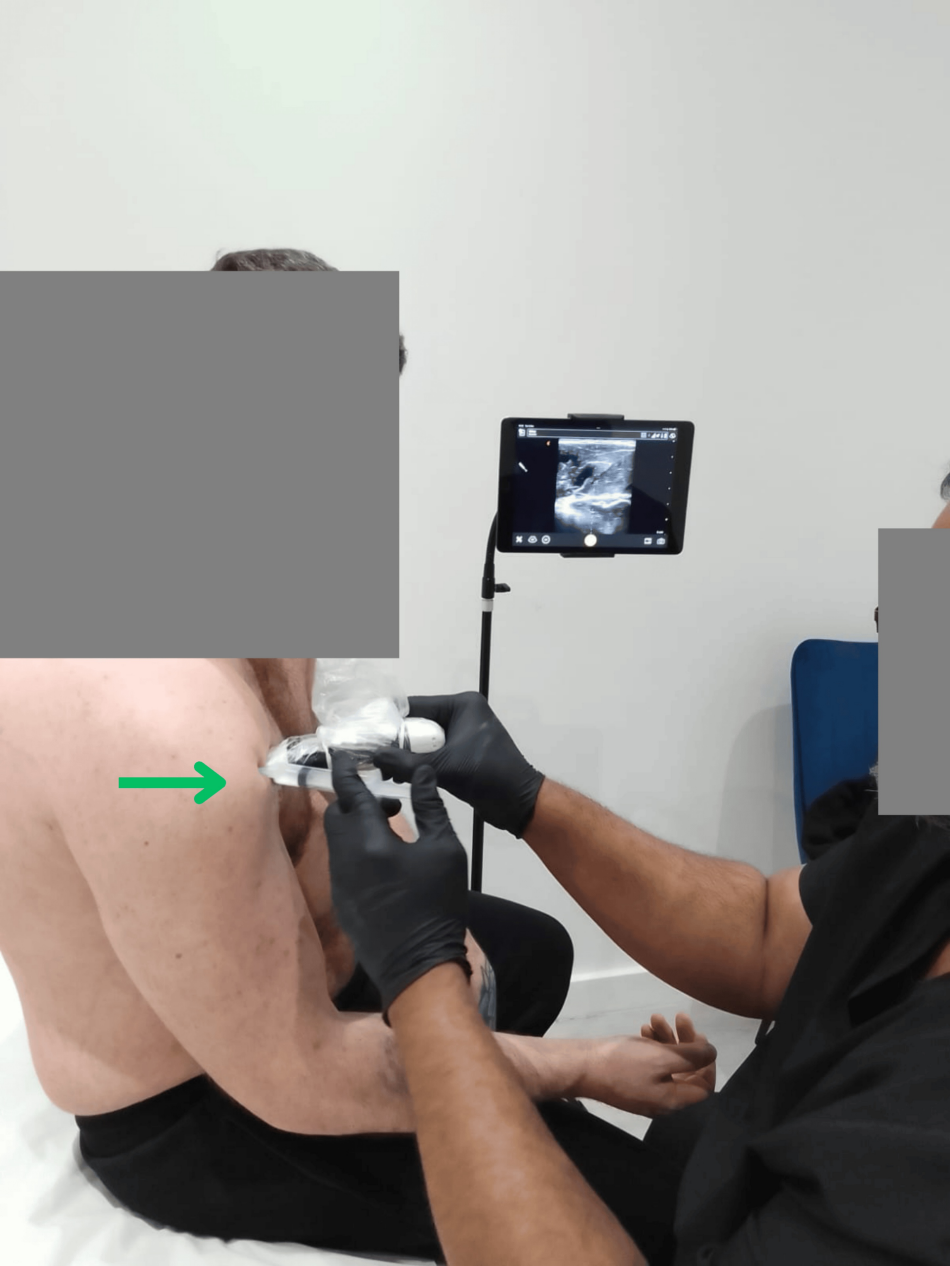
Real-time ultrasound-guided injection delivery of injectate (green arrow)
